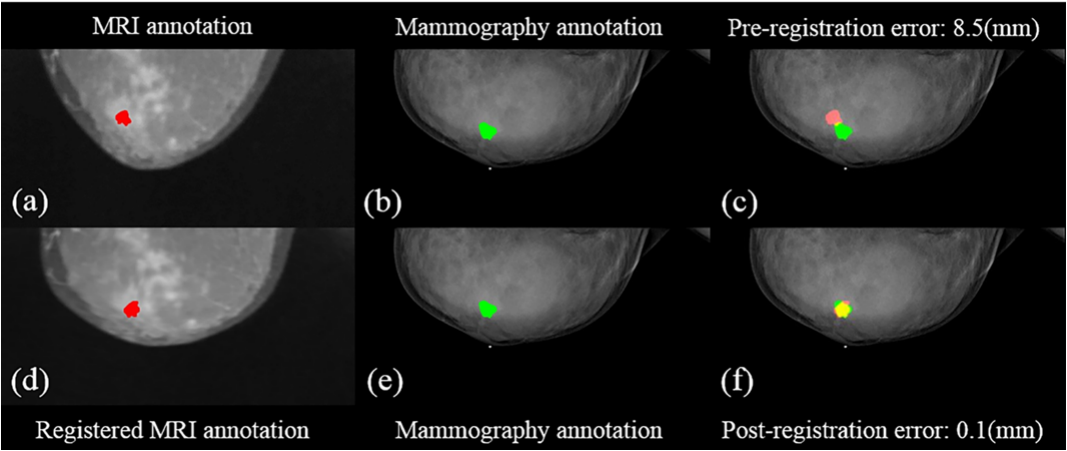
A 54-year-old woman with invasive ductal carcinoma in the upper outer quadrant of the left breast. (a) MRI annotation (b) the kinetic curve for MRI annotations indicating the Type 3 for the lesion (c) the deformed MRI annotation to register the mammography (d) the microcalcification observed in mammography in corresponding contour.
